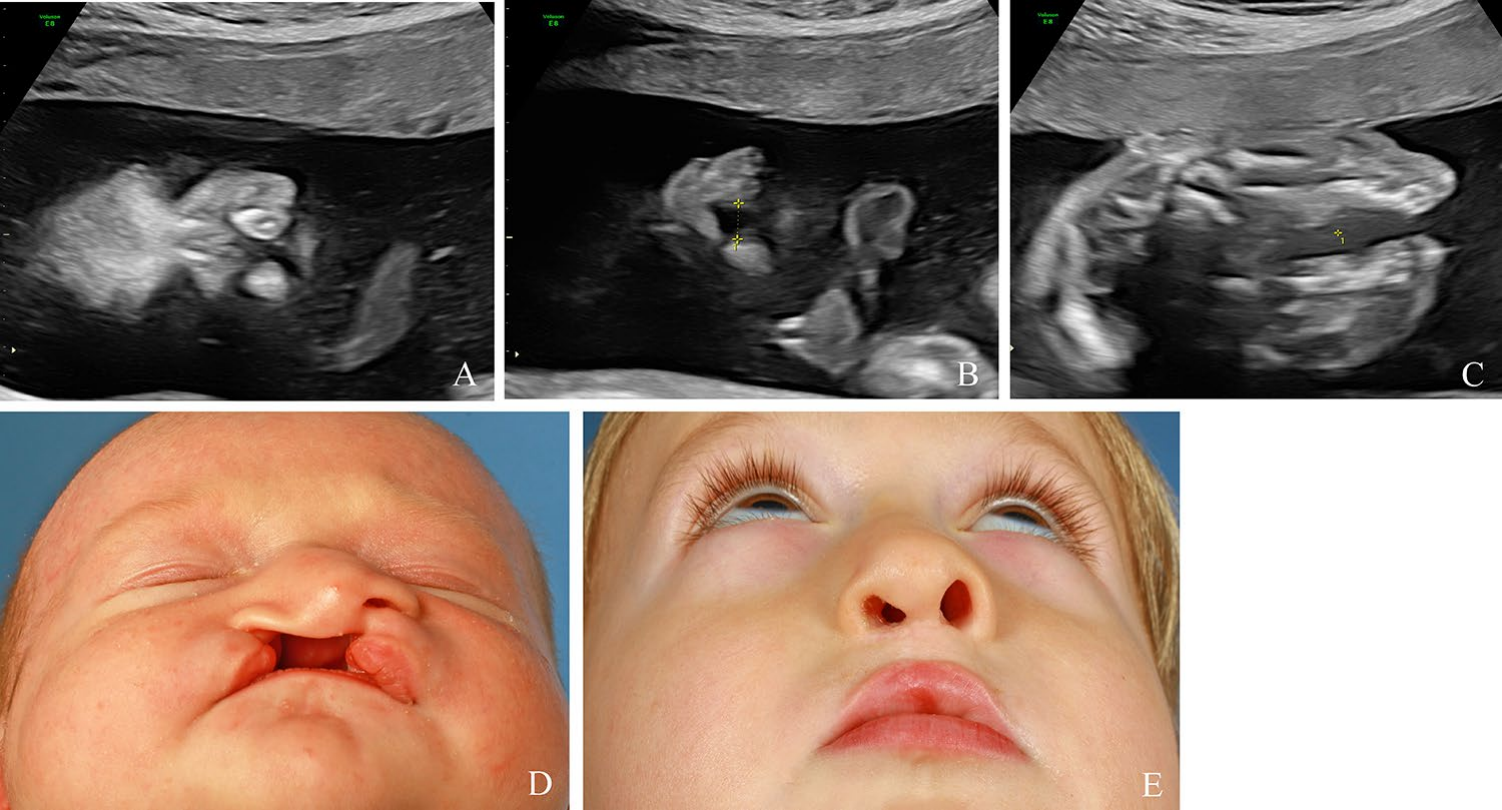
Midline hypoplasia and unilateral cleft lip and palate, visualized on ultrasound during the prenatal period in 2D, in frontal (A, B) and transverse (C) sections. Postnatal preoperative (D) and postoperative (E) result
